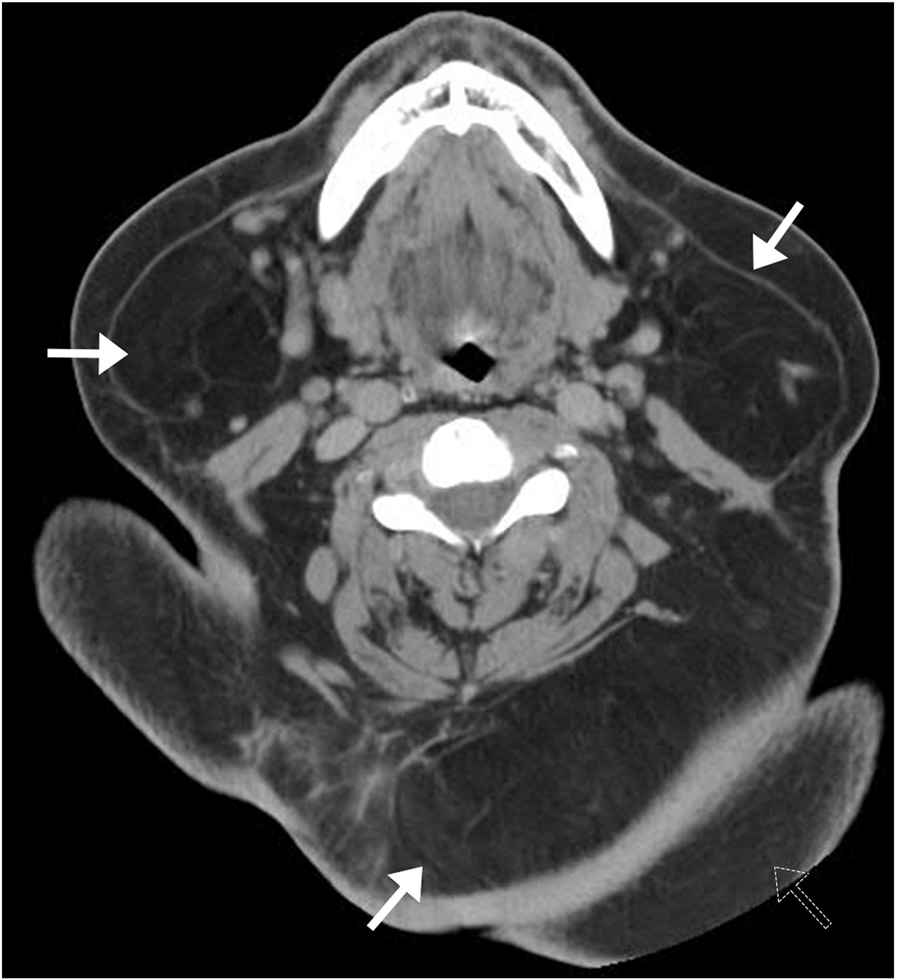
Madelung disease. Axial CT image of a patient with history of alcoholism presenting with shortness of breath demonstrates diffuse symmetric unencapsulated fatty accumulation of the neck (arrows) and upper back (dashed arrow). In the given clinical context, this is most consistent with Madelung disease or benign symmetric lipomatosis
